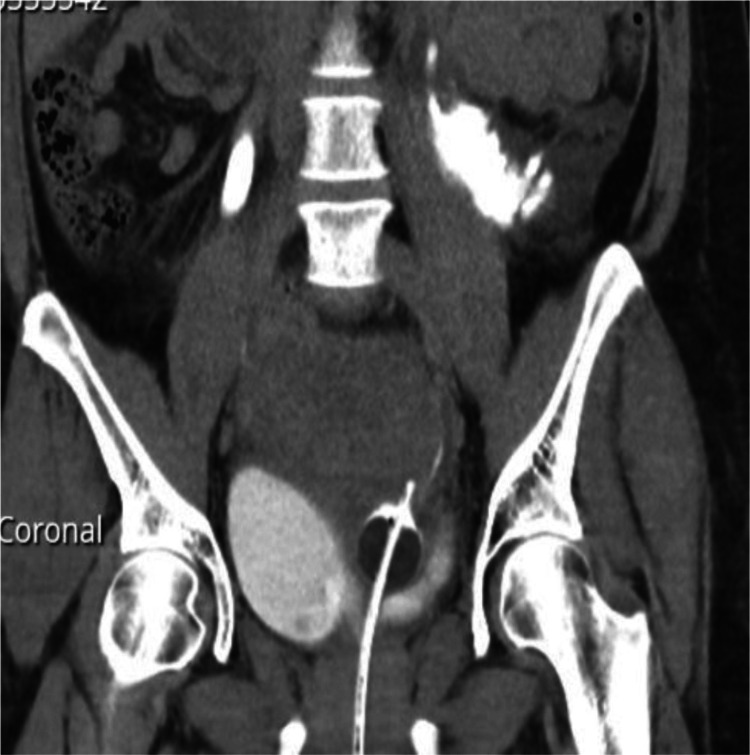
Coronal CT intravenous pyelogram demonstrating urinary catheter malposition, with catheter appearing to pass into the left distal ureter.
